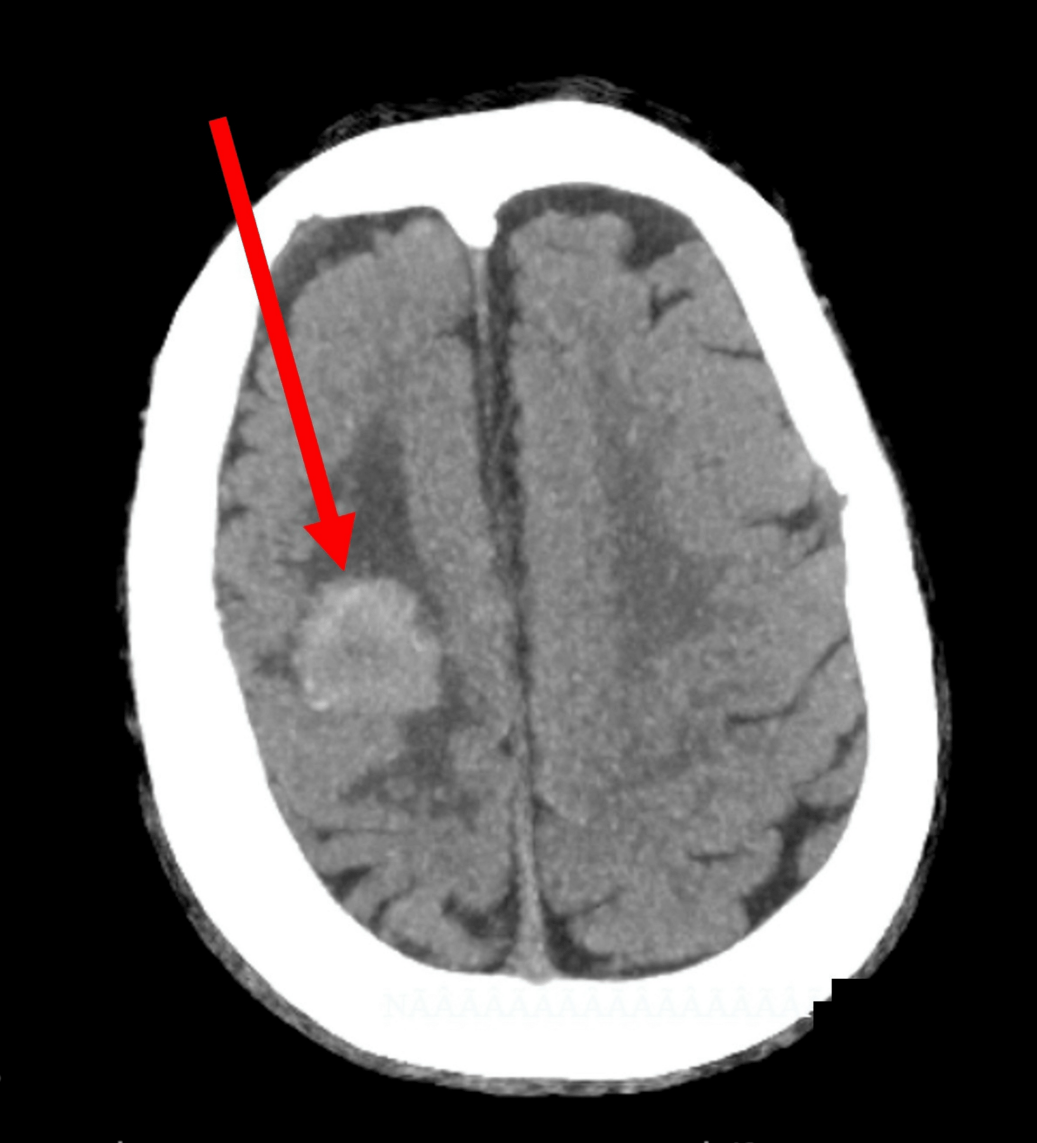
Axial brain CT scan The red arrow indicates an intra-axial lesion seen in the right frontal lobe, located at the precentral gyrus, oval-shaped, and isodense to gray matter, surrounded by vasogenic perilesional edema. It measures 26 x 20 mm in maximum diameter and is associated with a mass effect, partially compressing the right lateral ventricle CT: computed tomography
